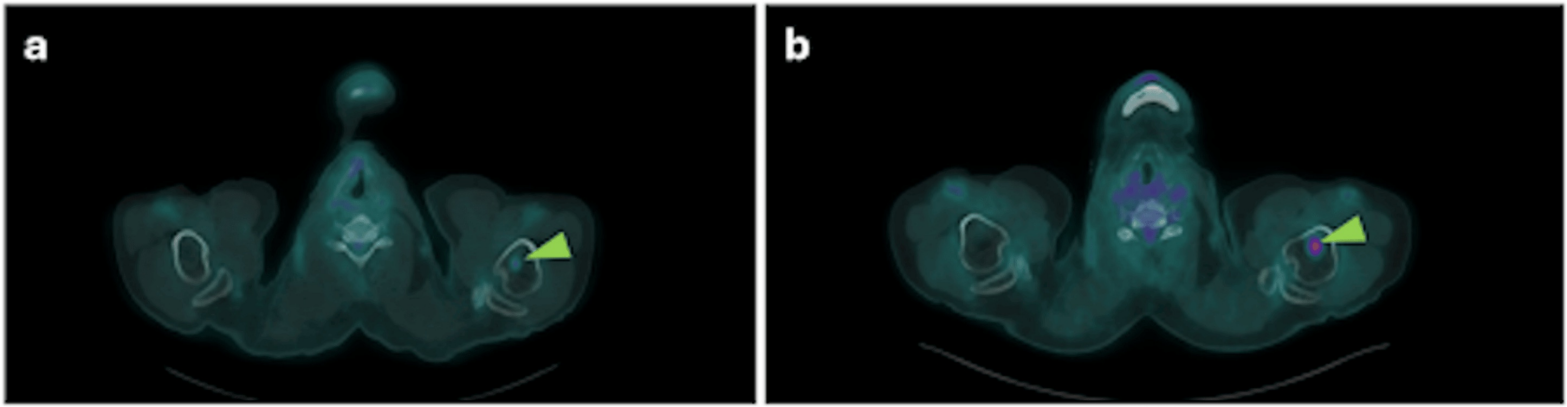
Axial FDG-PET/CT images from June 2023 (a) and July 2024 (b) demonstrate increased metabolic activity in a bone lesion in the proximal third of the left humerus (green arrowhead), compatible with disease progression. FDG: Fluorodeoxyglucose.
